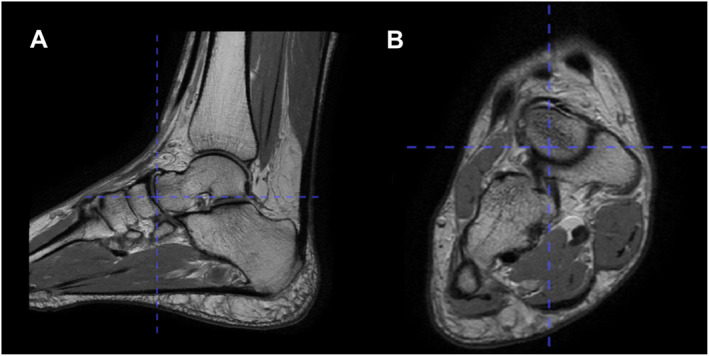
Sagittal and coronal plane MRI representing the location of interest. (A) Sagittal plane image with the blue cross‐hairs indicating the location of interest (talonavicular joint). (B) Coronal plane image with the blue cross‐hairs indicating the centre of the joint of interest. Both images (A) and (B) were aligned and correlated to ensure accuracy of measurement.
